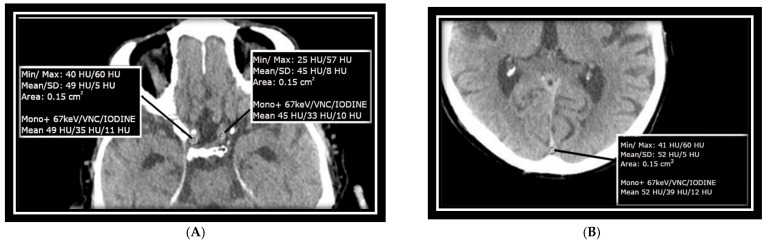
Axial non-enhanced CT scans of the neurocranium, highlighting two key areas: (A) bilateral ICA (internal carotid arteries): measurements were taken to capture the dimensions and structural integrity of the internal carotid arteries on both sides. (B) Confluence of sinuses: focus was placed on this region to assess the venous confluence at the base of the skull. Each measurement was conducted with meticulous attention to detail, ensuring that the measurements strictly adhered to the anatomical boundaries of the vessels and avoided any measurement errors on the vessel walls.
